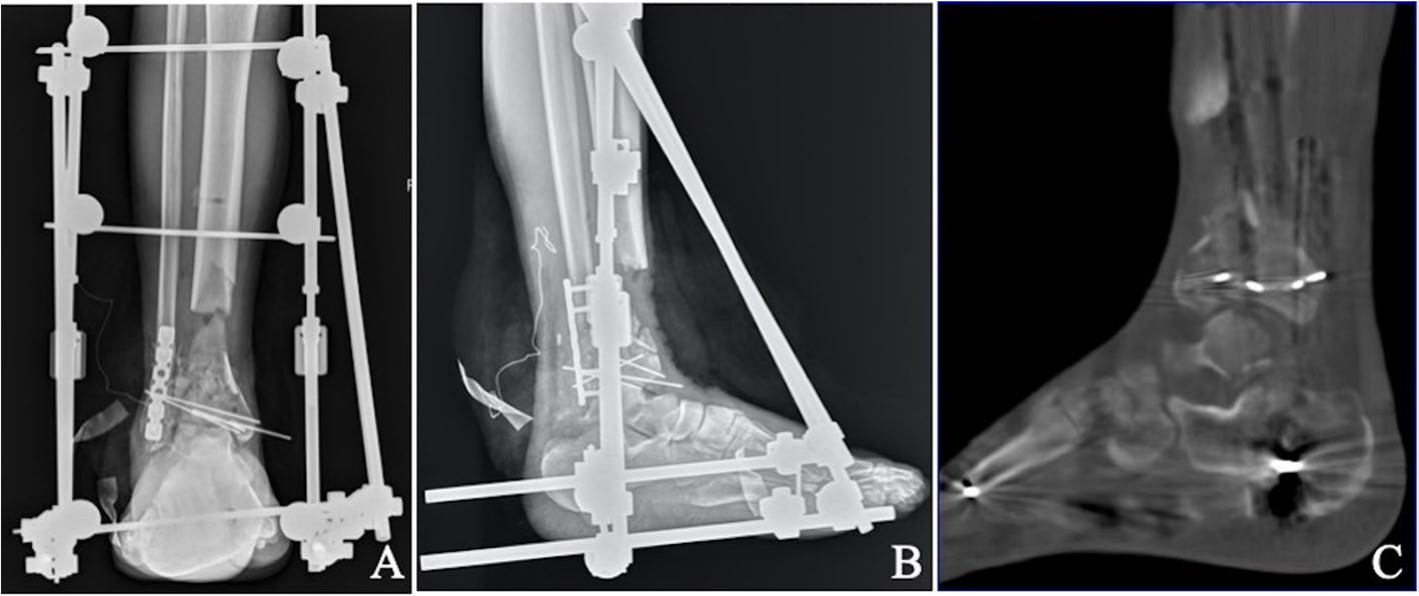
Anteroposterior (A & B) X -ray:after 15 days, thorough debridement and bone resection were done. The resulting defect was 6 cm in length. Limited ORIF was performed with K wires and fibular plate fixation was performed. Computed tomography (CT) scan (B): shows joint reduction, step or gap < 2 mm
